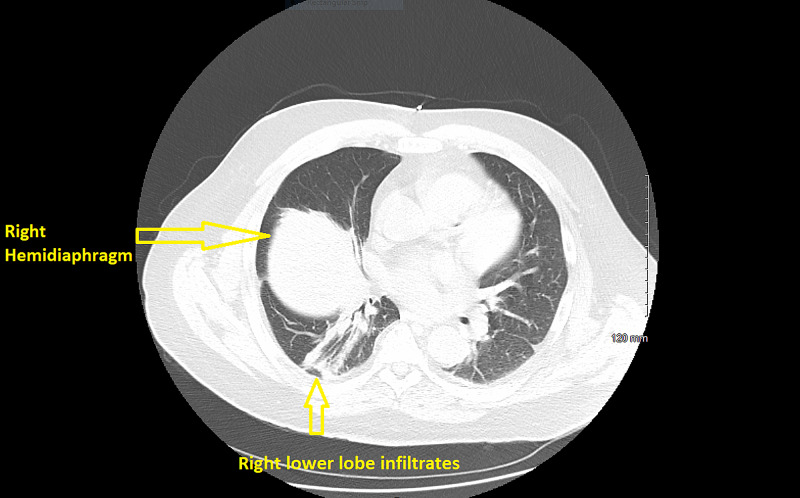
Axial section of the non-contrast CT chest demonstrating the right lower lobe infiltrates and elevated right hemidiaphragm.
